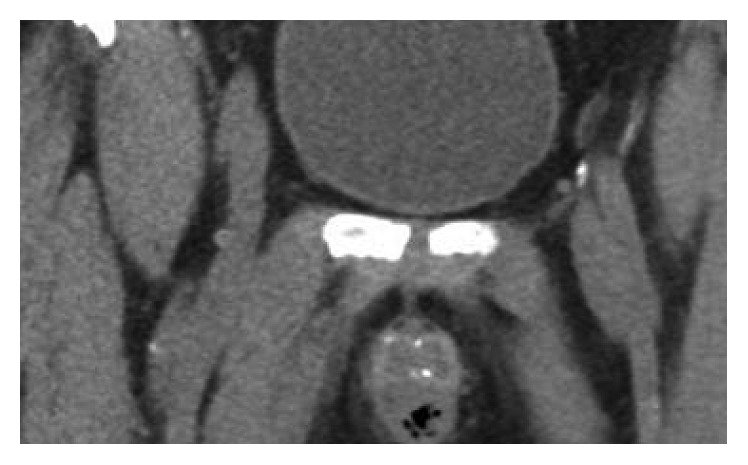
Coronal view of pelvic CT image demonstrating emphysematous changes in the bulb of the corpus spongiosum.
